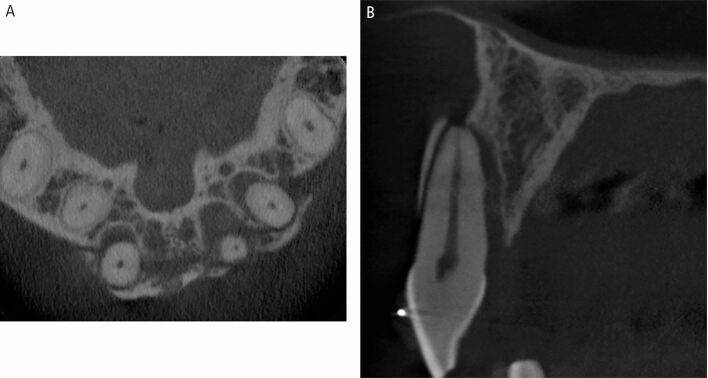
A CBCT scan of a dento-alveolar fracture extending over three maxillary incisor teeth, seen in the axial (A) and sagittal (B) planes
